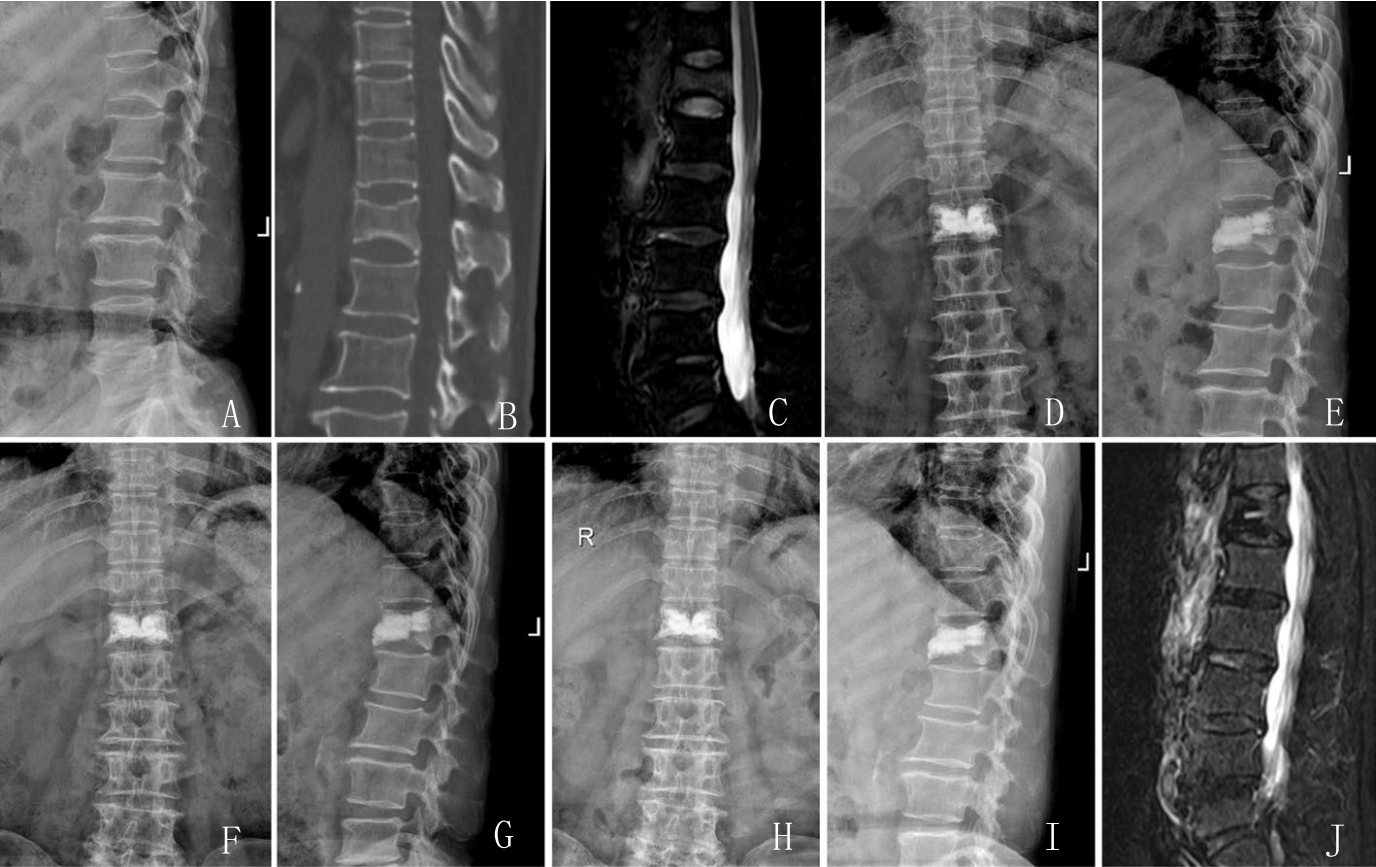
A 64-year-old female patient with OVCFs underwent PKP in June 2012. A-C Preoperative X-ray, CT, MRI showed fresh compression fracture of T12 vertebrae. D and E One day postoperative frontal and lateral spine radiographs showed that the cement was in place and well distributed in the T12 vertebral body. F and G Patients come to the clinic for review one month after surgery. H and I Patients come to the clinic for review one year after surgery. J At the last follow-up visit, MRI showed cement in place and no vertebral re-fracture
